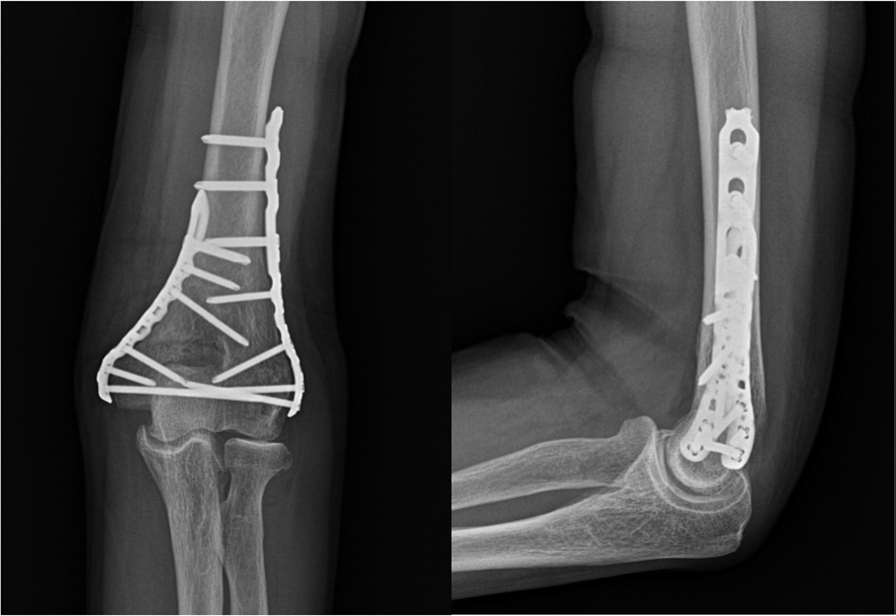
Post-operative radiographs of 75-year-old female patients. The plates are positioned such that the distal screws can be fixed parallel to the anterior surface of the humeral condyles. Two or three screws were fixed to the distal bone fragment on each side; additionally, efforts were made to implant one or more long screws on each side, for the opposite column to gain purchase
